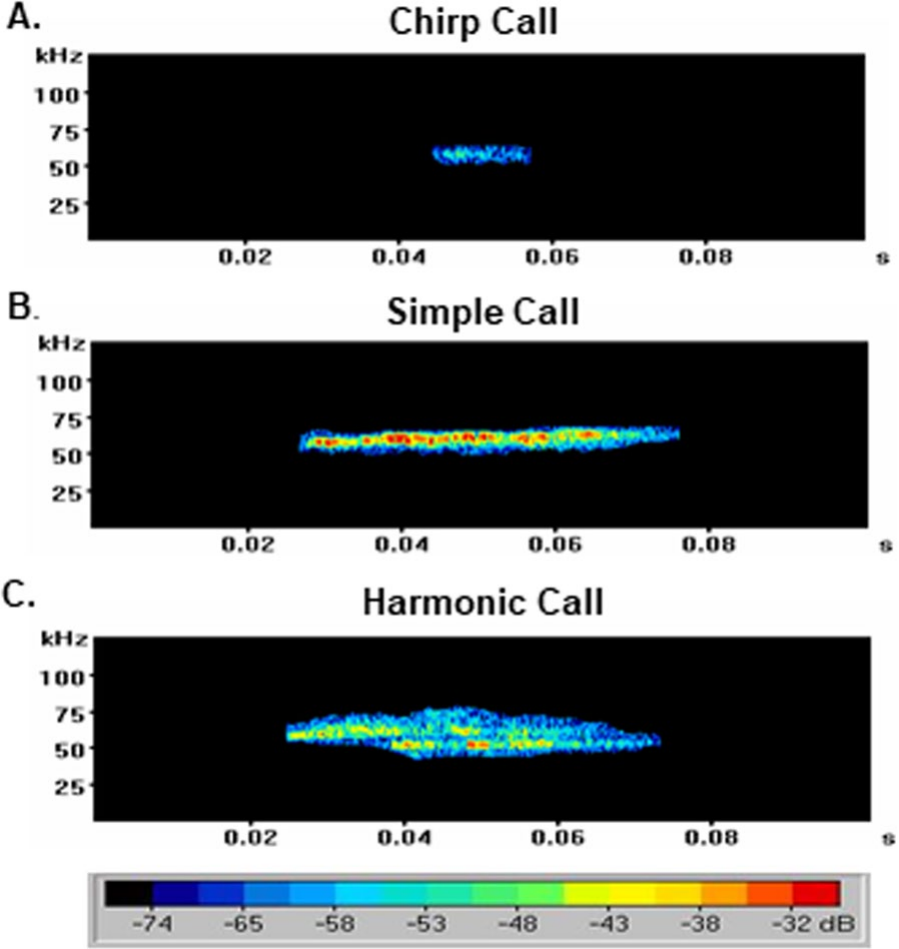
Representative spectrograms of ultrasonic vocalizations (USVs). USVs collected during a 5-min cage mate separation were analyzed. USVs were manually selected within spectrograms and categorized by call type. A Chirp calls were defined as low intensity USVs with a duration less than 0.02 s (20 ms). B Simple calls were defined as USVs longer than 0.02 s (20 ms) with “straight-line” intensity. C Harmonic calls were defined as a single USV with distinct tones of varying duration and intensity that create a “harmony”. Color-coded decibel level shown in key. kHz = kilohertz; s = seconds; dB = decibel
